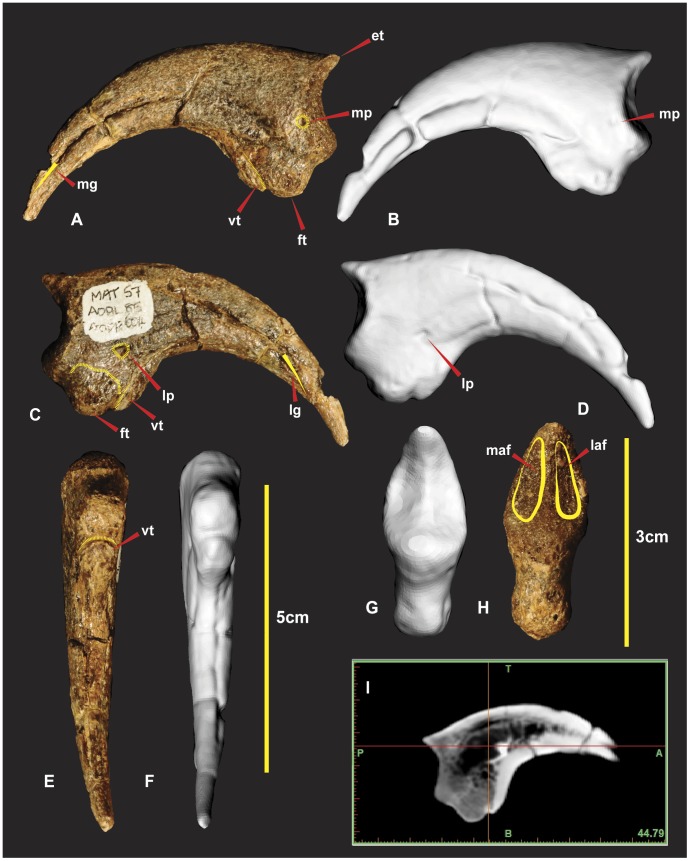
Right manual phalanx III-4. Right manual phalanx III-4 medial (A & B), lateral (C & D), ventral (E & F), proximal (G & H), central proximo-distal CT image (I).
